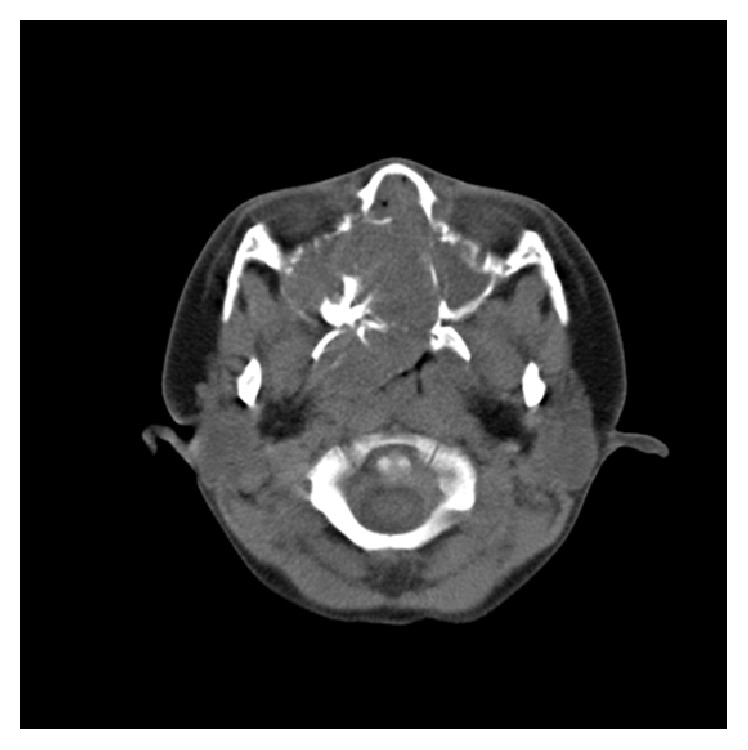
Precontrast axial CT scan shows a large round expansile mass hypoattenuating to the facial muscles and centered in the right maxillary sinus with plenty of calcifications.
